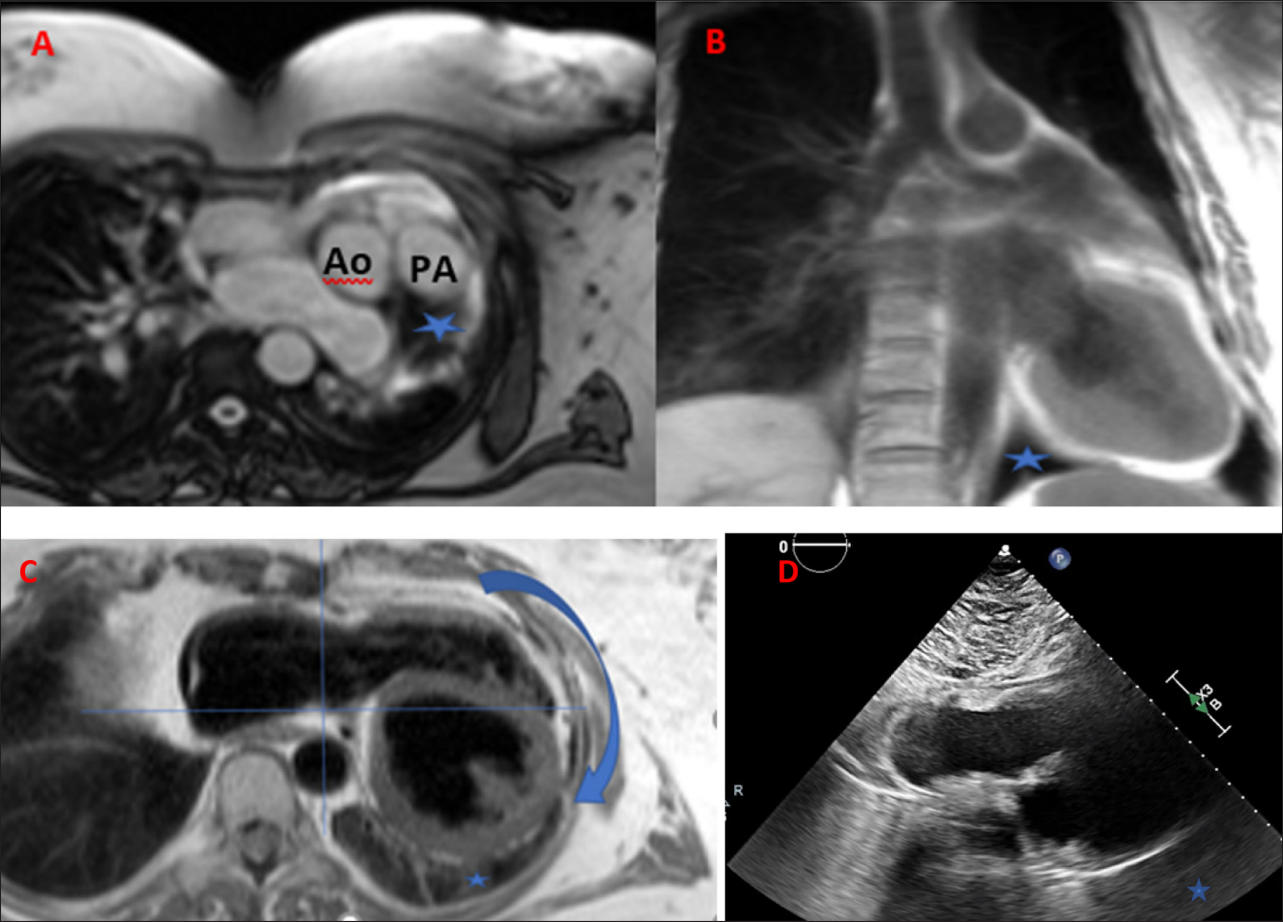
Cardiac magnetic resonance imaging of (A) axial localizer view and interposition of lung tissue between the main pulmonary artery and aorta (blue star), and (B) between the diaphragm and inferior left ventricle wall on coronal T2 weighted sequence. (C) HASTE (half fourier single-shot turbo spin-echo) sequence shows leftward position of the apex and loss of direct visualization of the left-sided pericardium as well as on the (D) subcostal transthoracic echocardiogram view. Ao: aorta; PA: pulmonary artery
